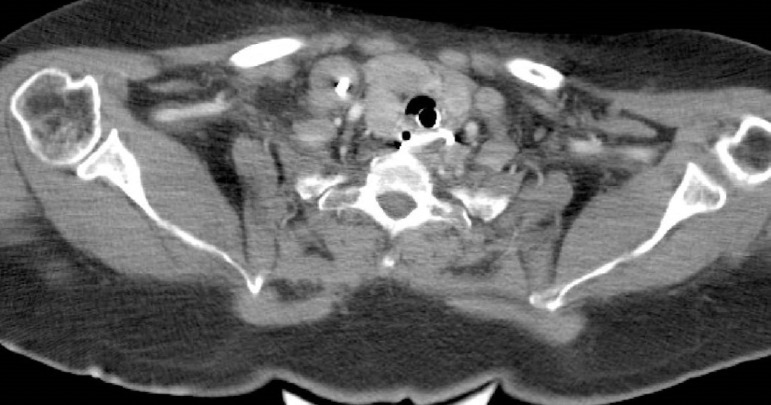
Computed tomography of the neck show a prevertebral shadow of an object with the density of a metal object.
